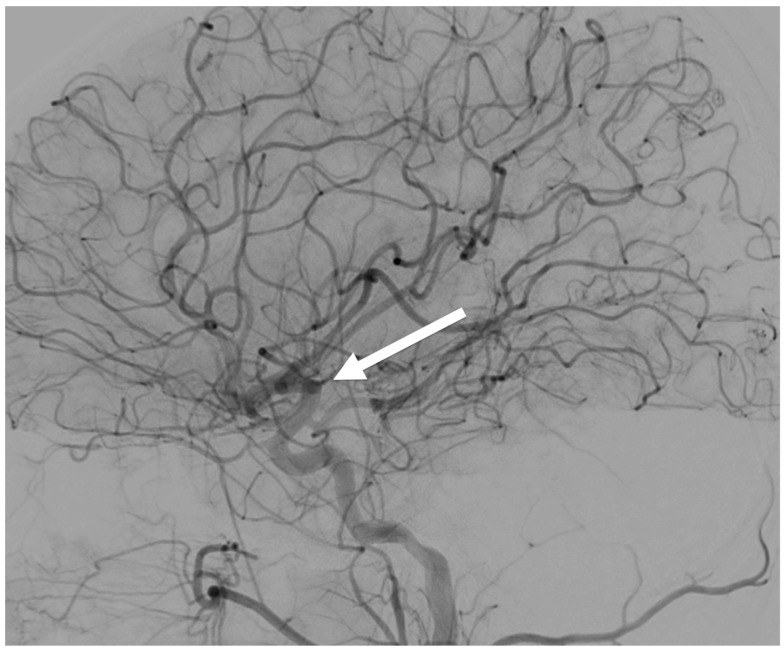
The in vivo angiography image from a 5 yr old pediatric patient that was obtained with the robotic scanner in CATHLAB mode. The data acquisition in the CATHLAB mode reached acquisition rates up to 10,000 Fps as the detectors rotated around the head. The CT 3D morphology of the tissue of the total organ and of the system of arteries and veins was reconstructed using the 3DNet vascular filter [51]. Once the 3D space was assigned, the dynamic tissue deformation and the blood flow data could be visualized synchronously.
